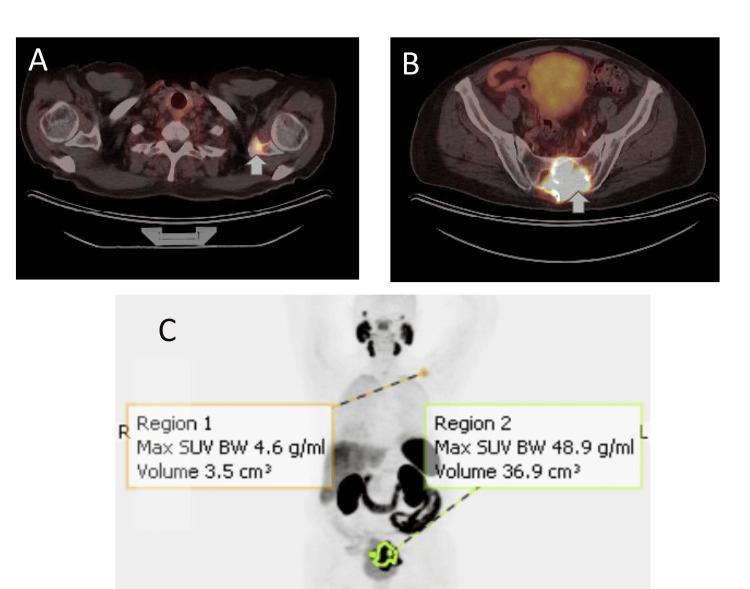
PET-CT scan axial images reveal (A) hypermetabolic sacral mass, (B) hypermetabolic left scapular lesion, and (C) whole body images redemonstrating hypermetabolic regions in the left scapula and sacral region.
